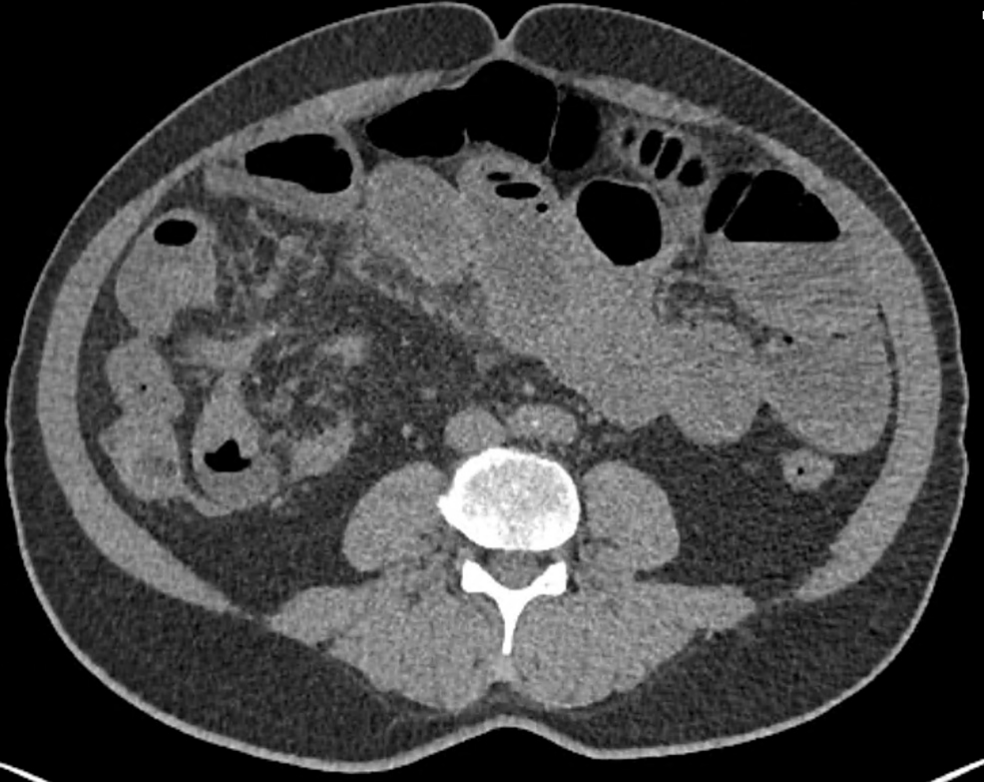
Axial non-contrast CT KUB image CT: computed tomography; KUB: kidneys, ureters, and bladder
